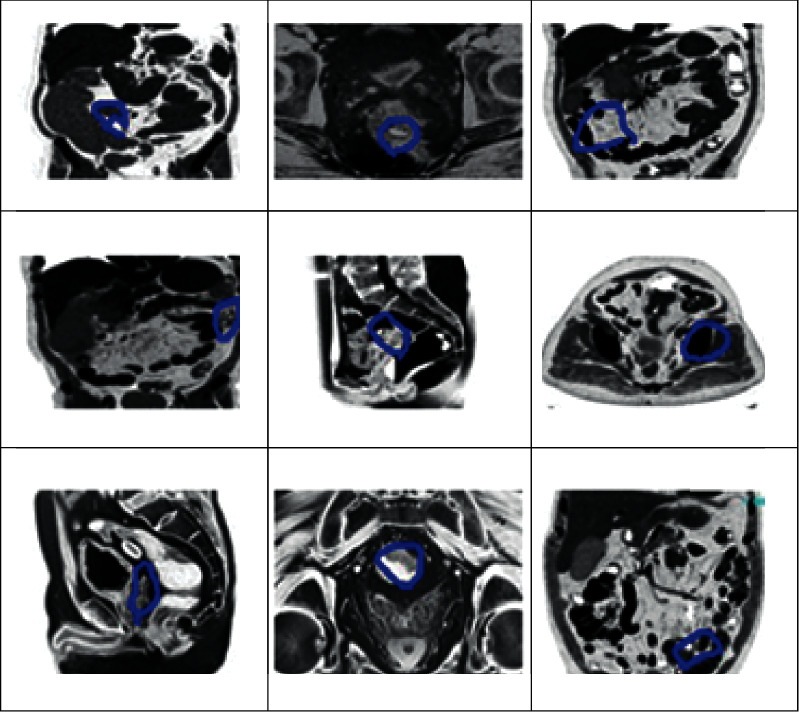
Sample colorectal cancer MRI images.
